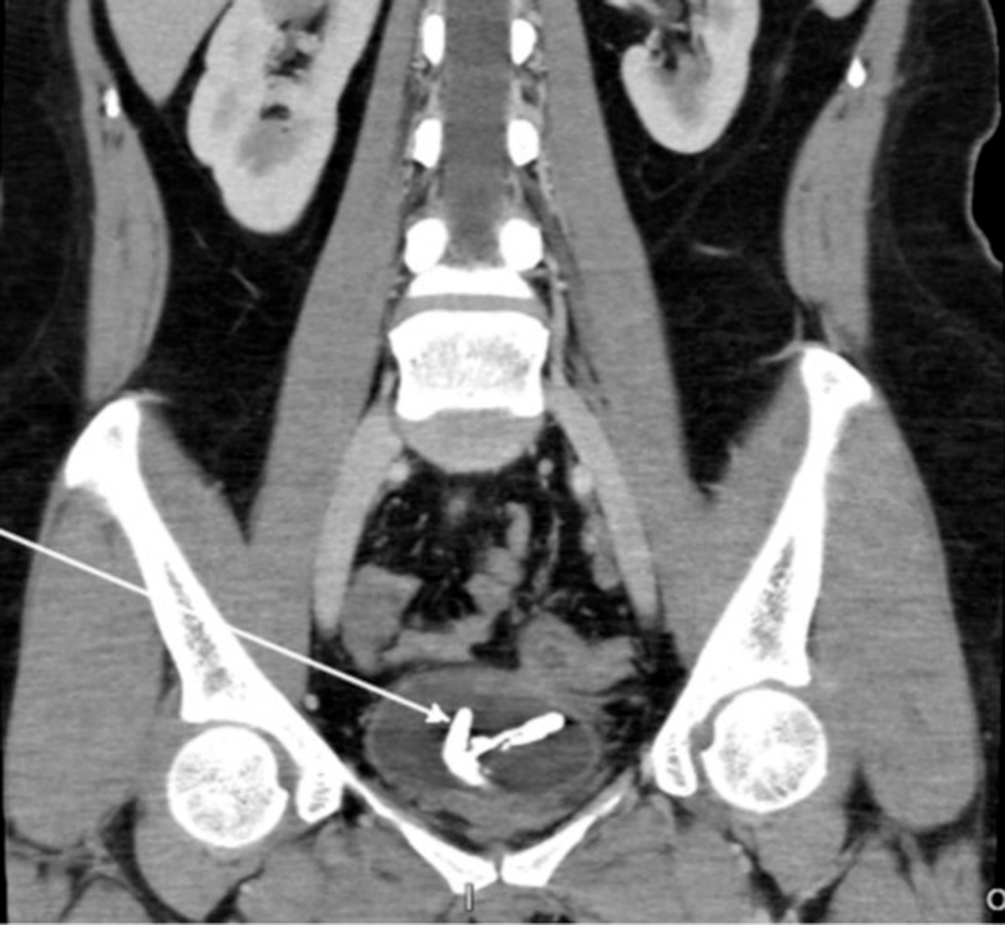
CT scan revealed an encrustation of IUD in the bladder.
